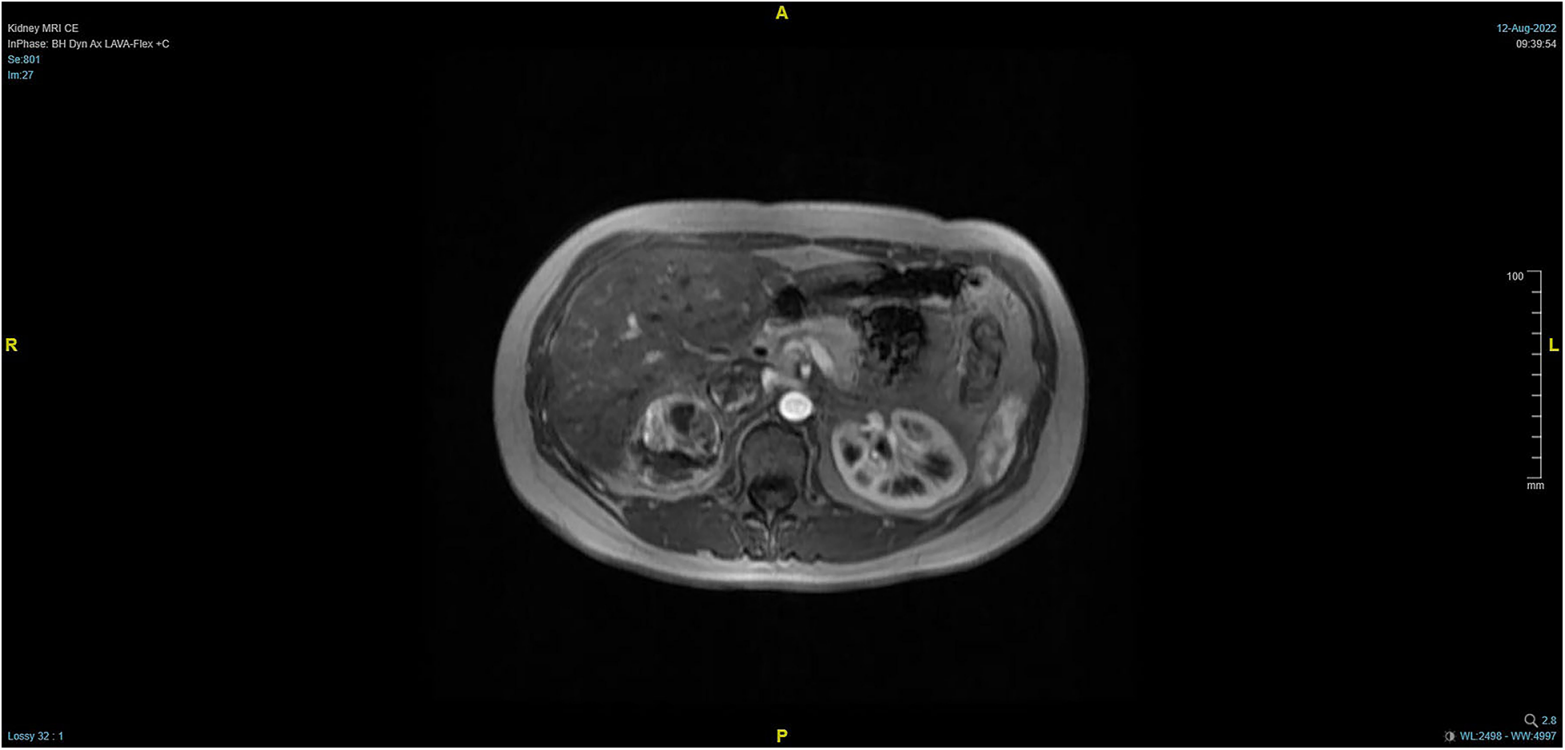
Pre-operative MRI showing that the tumor lesion enlarged upward along the right renal vein into the inferior vena cava (coronal planes).
